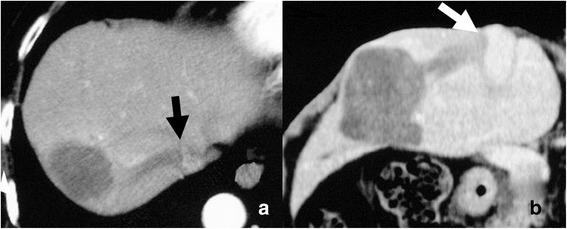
CT after three sessions of hepatic arterial infusion chemotherapy shows marked shrinkage of the main tumor and tumor thrombus. Additionally, the tip of the tumor thrombus (*arrow*) can be confirmed around the orifice of the right hepatic vein. a View in the axial plane. b View in the coronal plane
